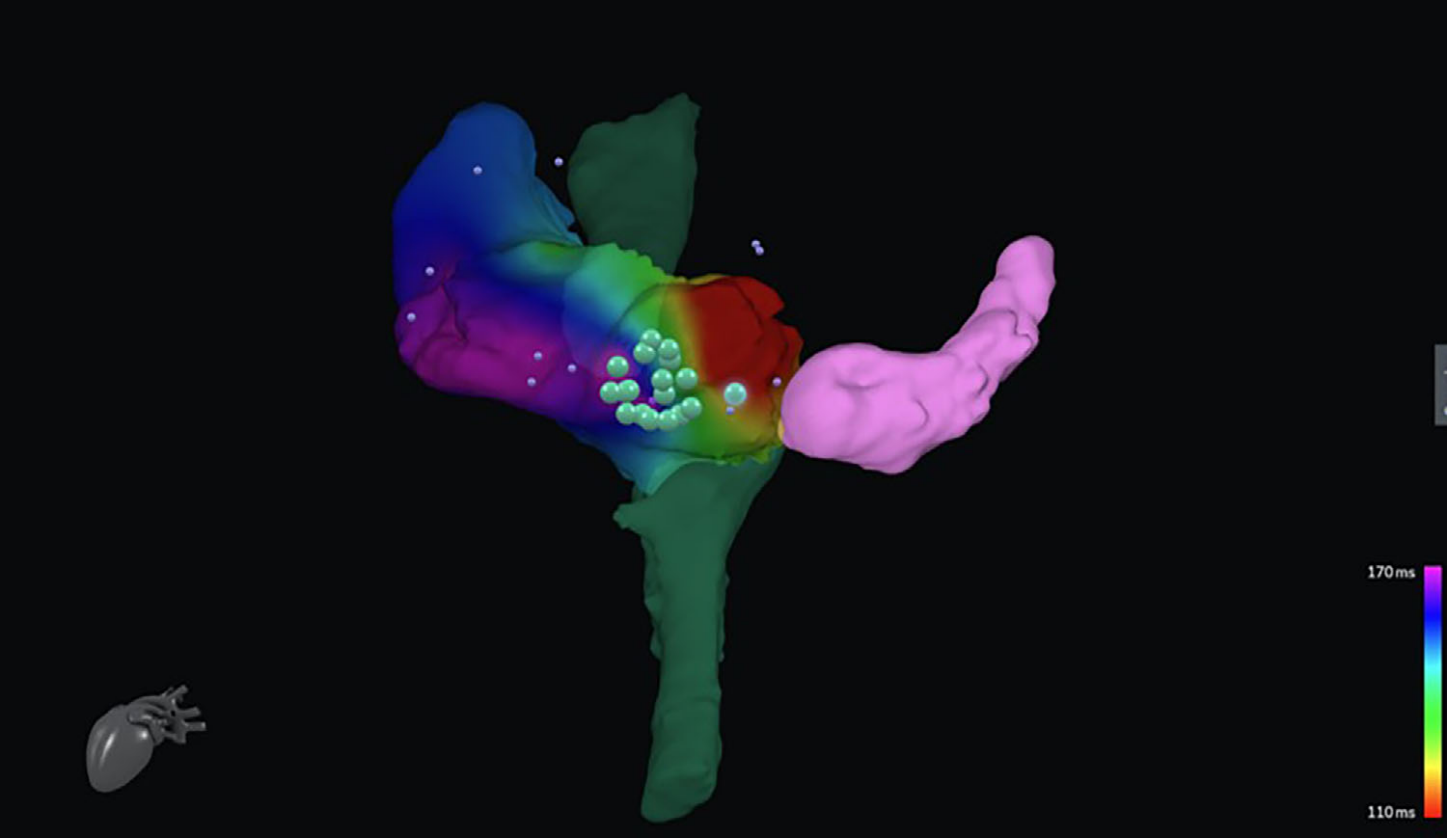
Real‐time CMR‐guided atrial flutter ablation. The use of a dedicated software allows for the merging of three‐dimensional anatomical reconstruction from MRI images and electrophysiological data. The inferior and superior vena cava are depicted in dark green, while coronary sinus in pink. An electro‐anatomical map is applied to show the activation pattern of the right atrium after the ablation. Big green spots represent the ablation points on the cavo‐tricuspid isthmus. Little pink spots represent mapping points.
